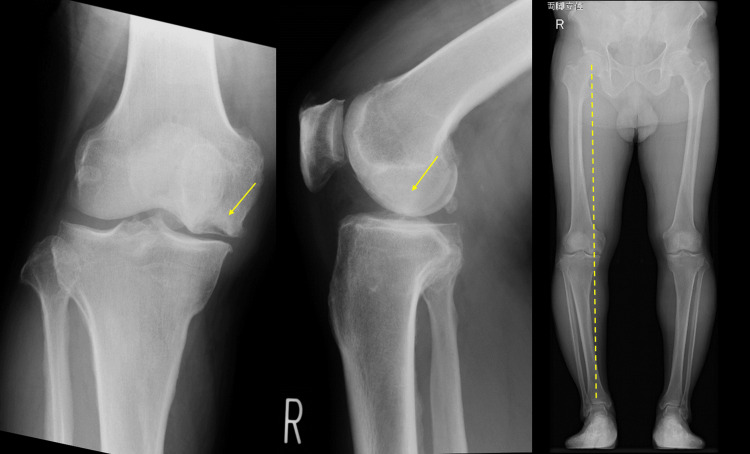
Preoperative radiograph of the right knee The arrows indicate bone and cartilage defects. The dashed line is the Mikulicz line.
